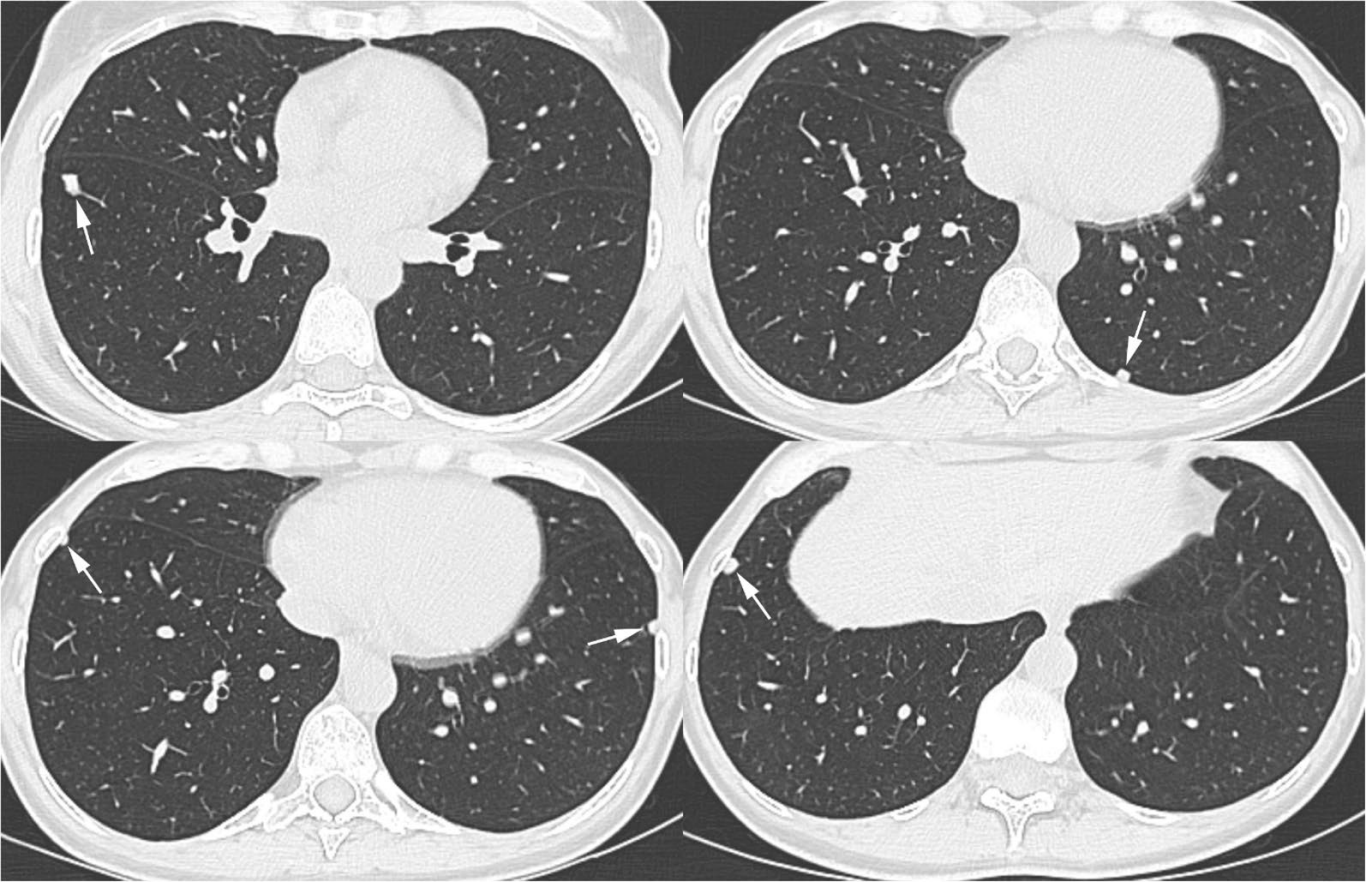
Axial thin-section CT images with lung window setting show multiple peripheral pulmonary nodules (arrows) in both lower lobes
